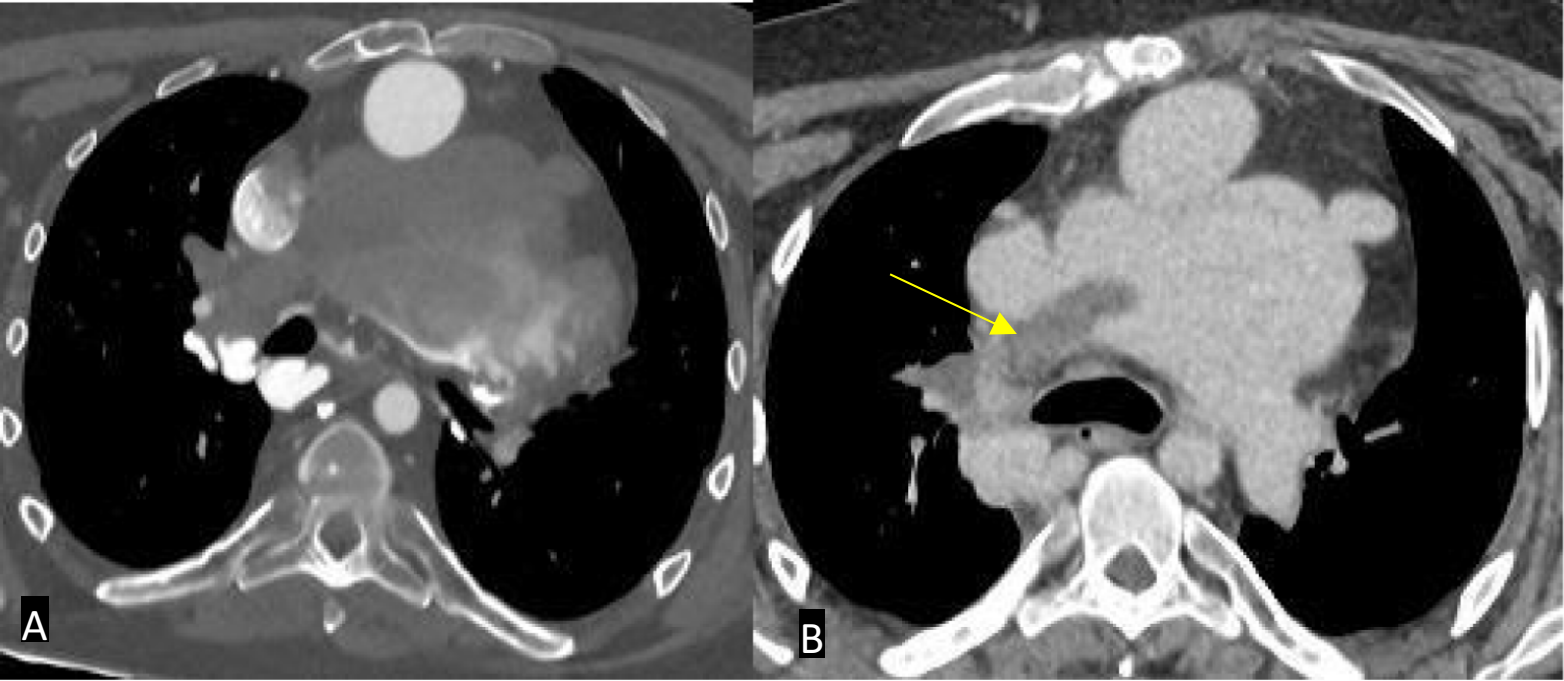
Axial CT images in a patient with an extra-cardiac conduit Fontan circuit. A Inadequate protocolling which resulted in suboptimal opacification of the circuit. Note that it is impossible to identify a true thrombus. B A single contrast phase, delayed phase acquisition protocol was subsequently used in the same patient which opacified the Fontan circuit adequately and allowed identification of a thrombus in the right main pulmonary artery (yellow arrow)
